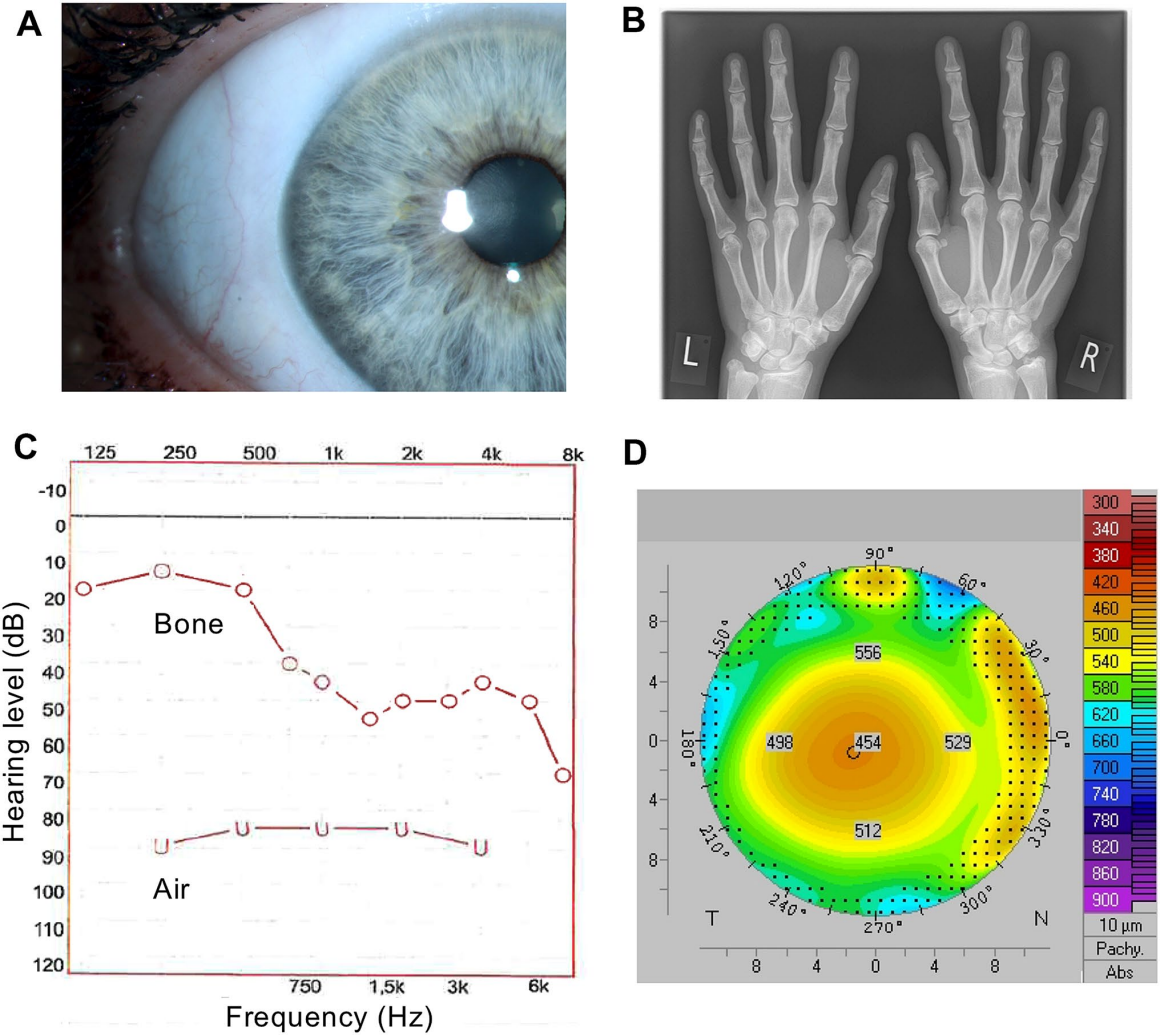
Clinical phenotype. a Blue sclera exemplary shown for patient II.1 (Slit lamp photograph). b Hand radiograph demonstrating long fingers. c Audiometry showing a mixed sensorineural and conductive hearing loss. d Corneal pachymetry showing moderately reduced corneal thickness
